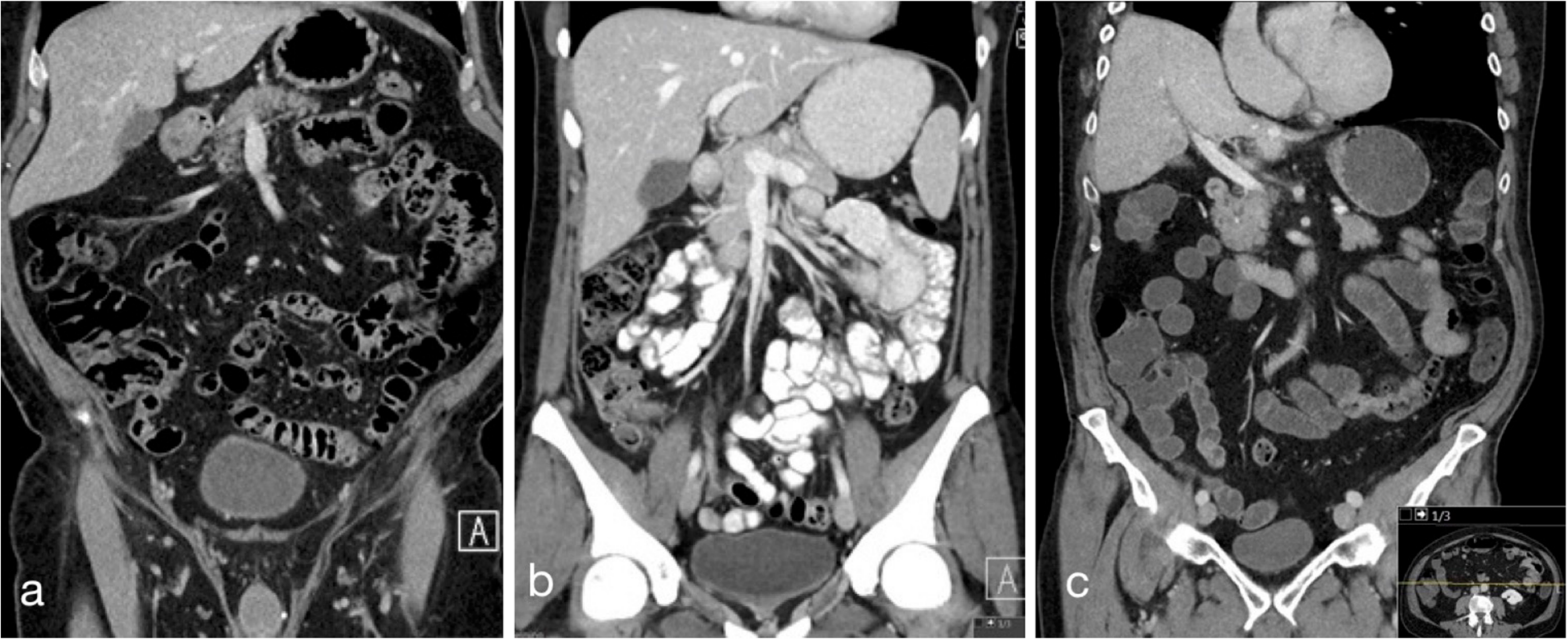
Abdominal computed tomography coronal view after oral administration of Lumentin 44 (a), diluted Omnipaque (b), and MoviPrep (c). Standard abdominal window-setting (window width 400 HU, window level 60 HU)
